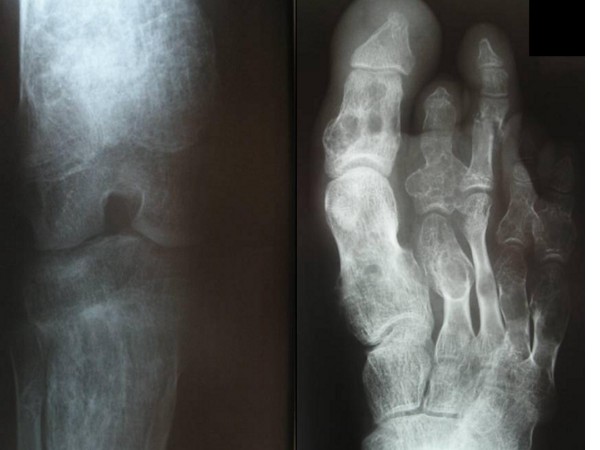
Anteroposterior lower limb radiograph of the distal femoral, proximal tibial showed enlarged metaphysis near the knees associated with striae of fibro-chondromatosis. The foot radiograph showed macrodactyly and severe deformities associated with dysplastic 2^nd^, 4^th ^and 5^th ^metatarsals respectively. Note transverse fracture (secondary to minimal trauma) over the proximal phalange of the 3^rd ^toe.
